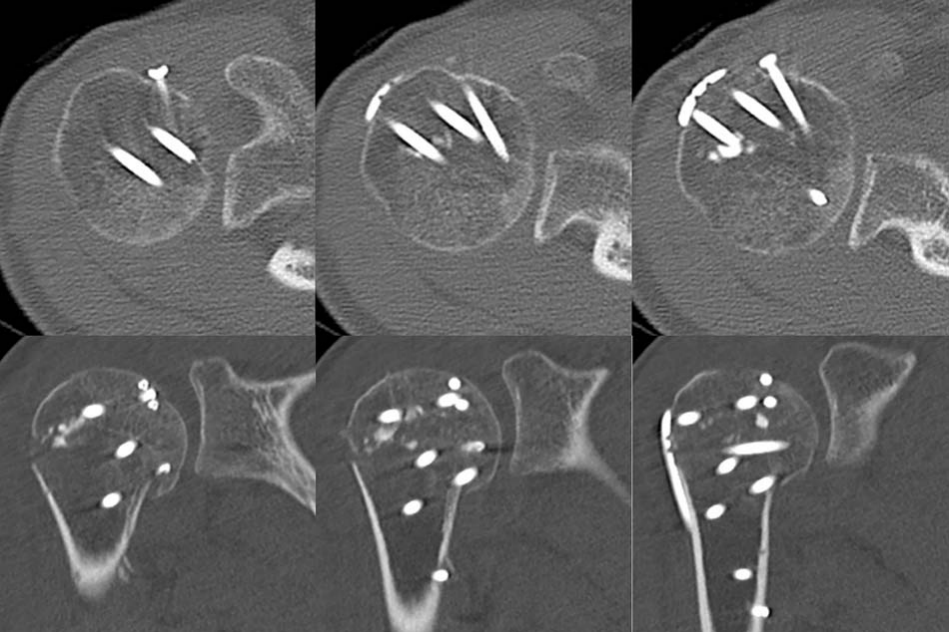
Two years later, pre-implant removal CT showed that the reduction in the depressed surface had been maintained with no obvious arthritic changes.
